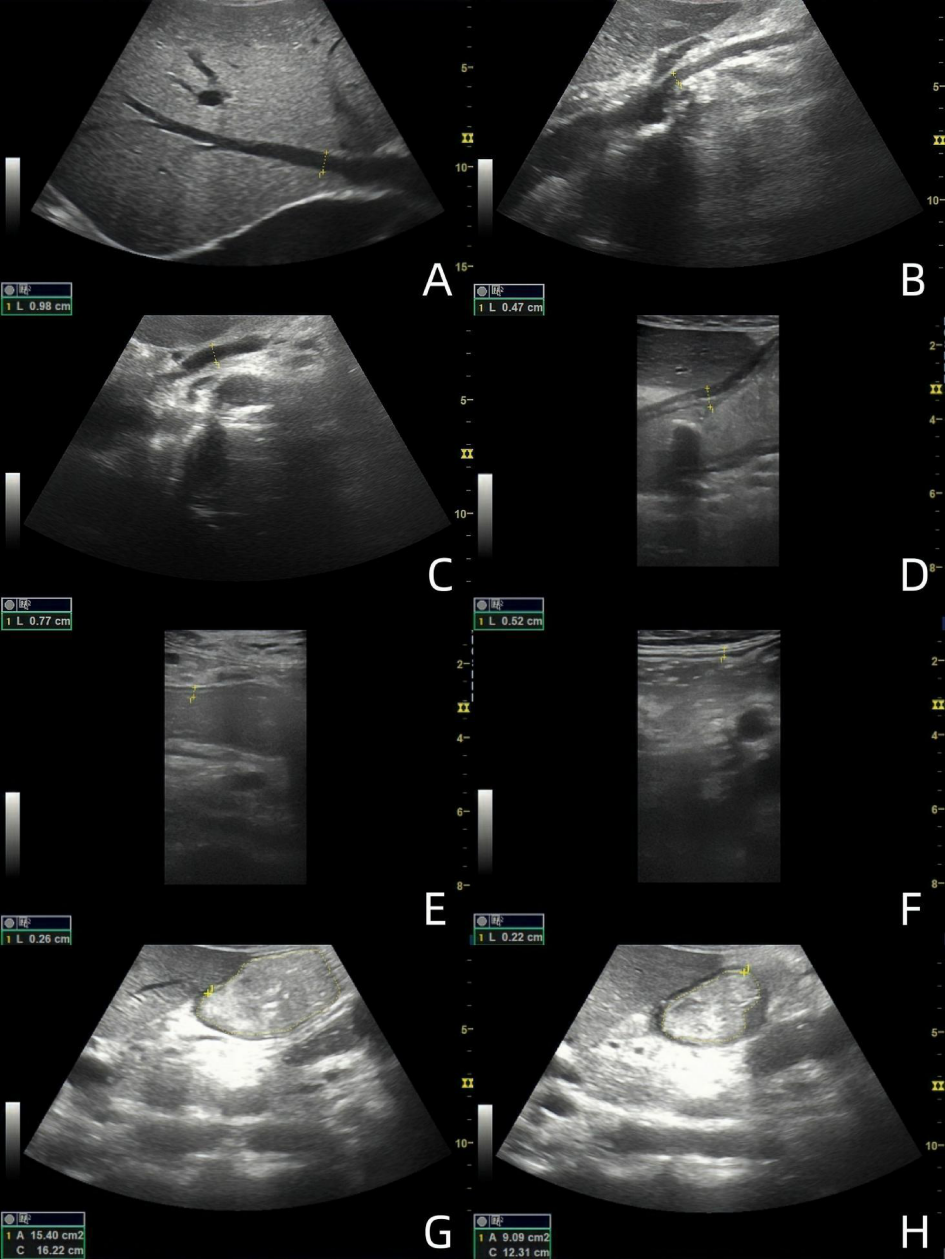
Ultrasound images of 49-year-old male patients with acute heart failure. (A) Maximum diameter of hepatic vein; (B) SMA inner diameter; (C) SMV inner diameter; (D) antral wall thickness; (E) Jejunal wall thickness; (F) Ascending colon wall thickness; (G) Maximal diastolic area of gastric antrum; (H) Minimal contraction area of gastric antrum. (The images are sorted from left to right and from top to bottom as A-H).
